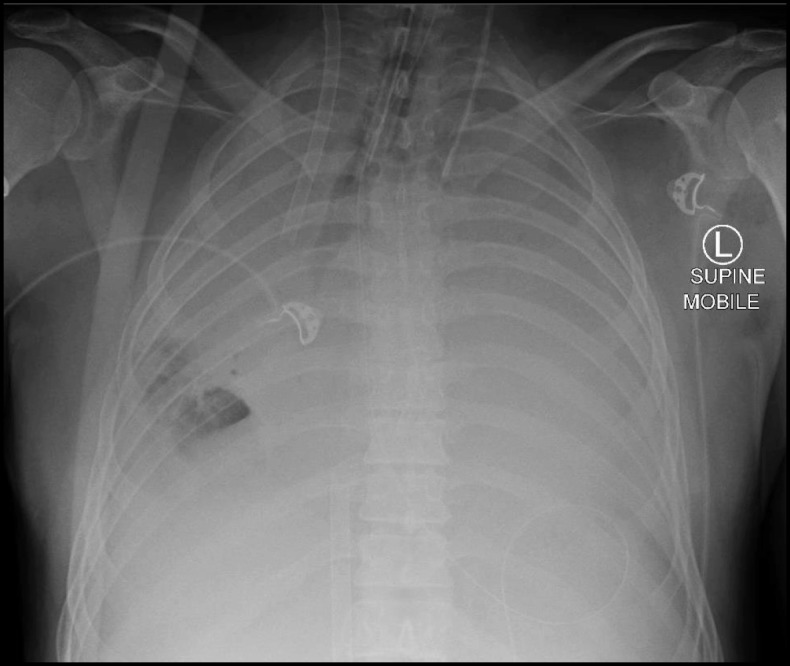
Interval plain supine anteroposterior chest x-ray demonstrating complete bilateral lung collapse three days after presentation and one day after the commencement of extra-corporeal membrane oxygenation
